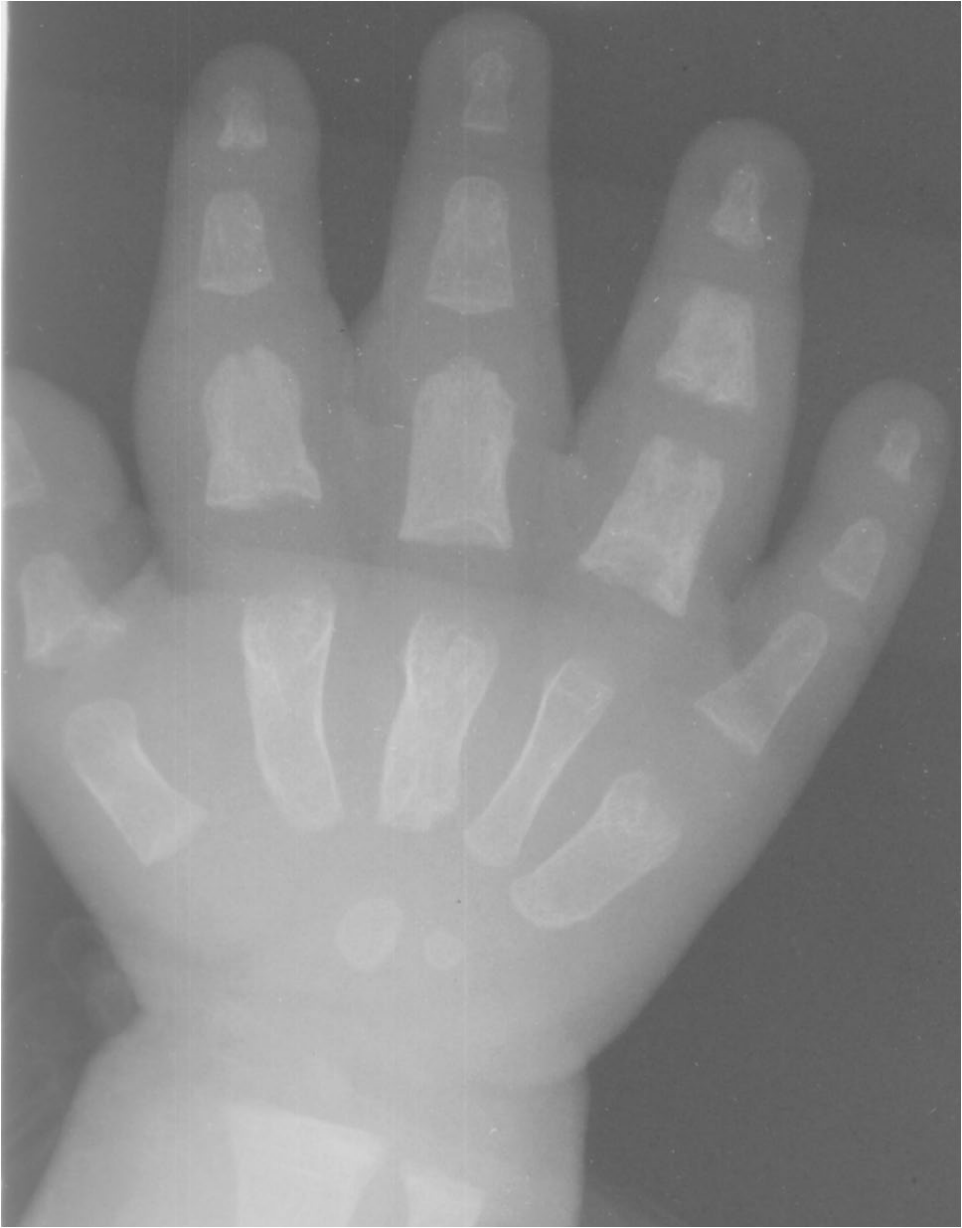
Dorsopalmar radiograph of the right hand in a 9-month-old girl, presenting with a painless swelling of the fingers for 2 months. There is soft tissue swelling of the second through fourth digits with fusiform expansion of the second, third and fifth metacarpals and fusiform swelling and erosion of the first, second, third and fourth proximal and fourth middle phalanges, typical of tuberculous dactylitis (spina ventosa)
